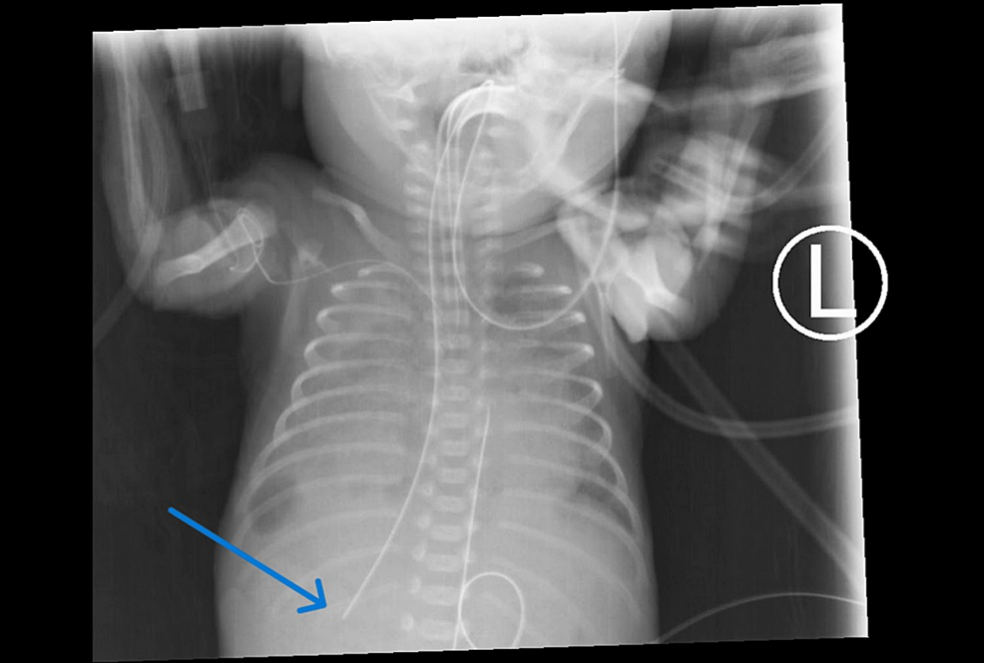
Abnormal oral gastric tube positioning on a chest radiograph. The tube can be visualized as coursing down on the right side of the patient in an inferolateral fashion with termination in the area of the right lung base. This could possibly indicate abnormal anatomy or, as in this case, abnormal positioning of the oral gastric tube.
